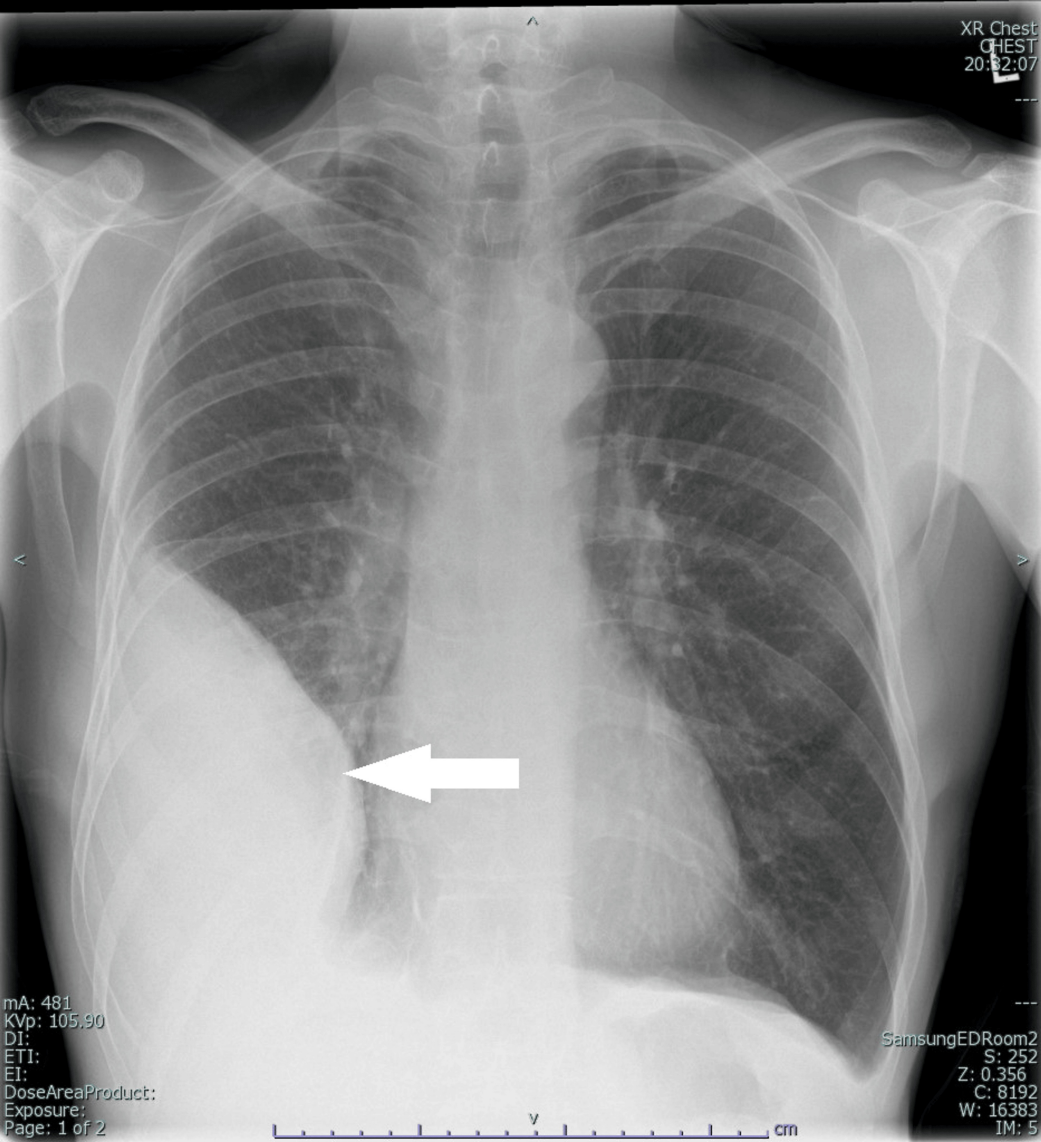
Arrow in chest X-Ray shows a large subpulmonic collection within the right mid to lower zone.
